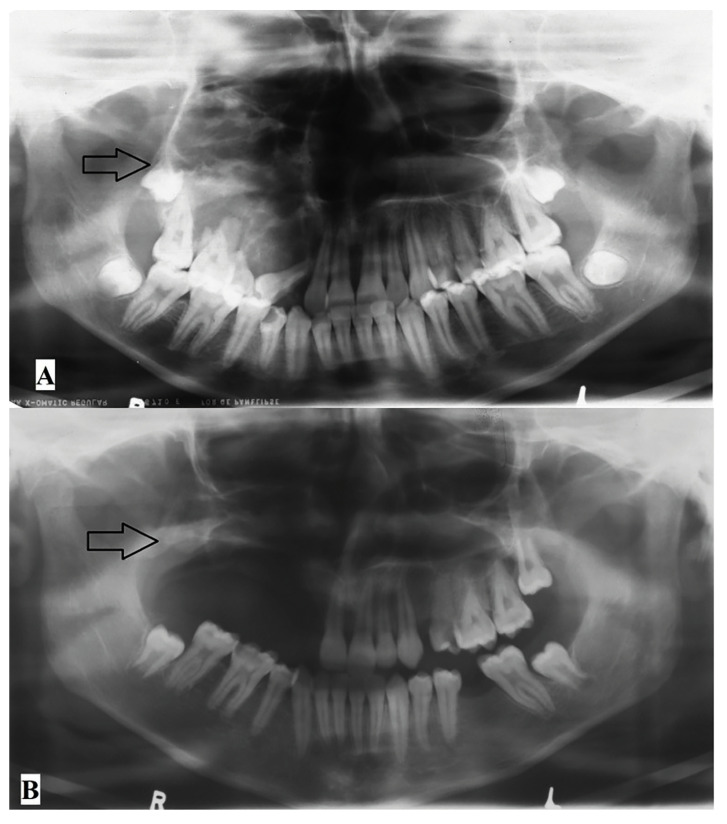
A: Pre-operative panoramic radiograph showing JTOF in the right posterior maxilla. B: Follow-up panoramic radiograph of the same patient three years post curettage with peripheral ostectomy showing no sign of recurrence of the lesion
